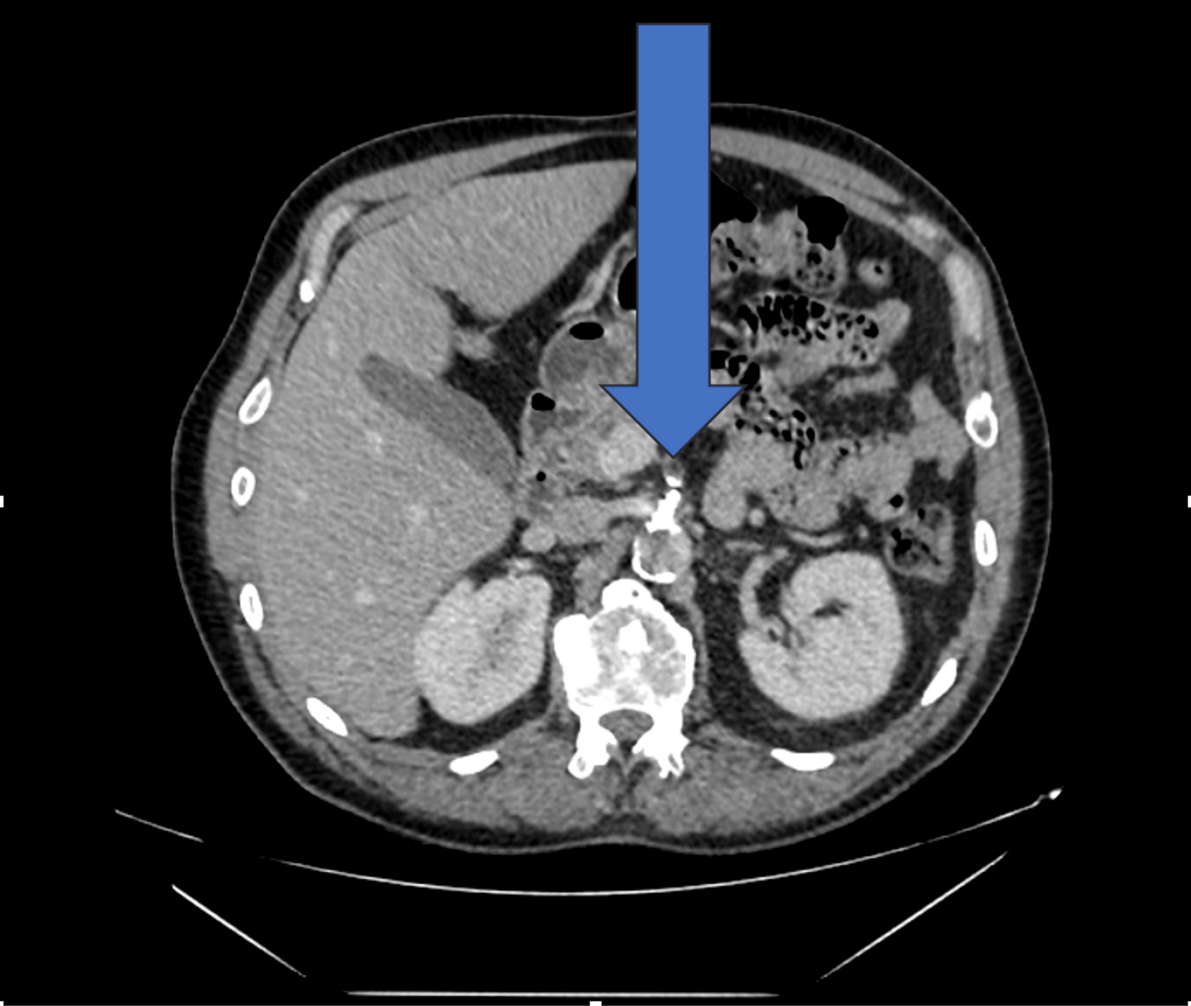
CECT abdomen and pelvis. The arrow indicates the proximal superior mesenteric artery, showing near-complete occlusion with a calcified, narrowed lumen CECT: Contrast Enhanced Computed Tomography
